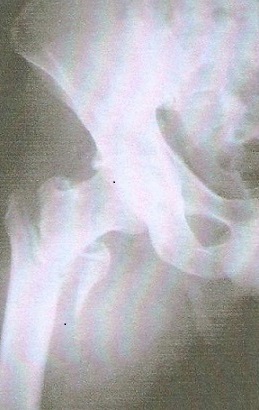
Radiographie de hanche droite de face montrant une fracture per-trochantérienne complexe du côté droit
